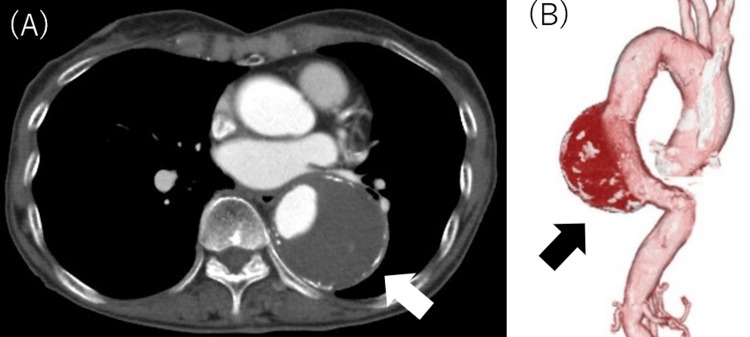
Preoperative contrast-enhanced CT. (A) Short-axis image revealing a 60 × 52 mm aneurysm with an abundant mural thrombus (white arrow). (B) A 3D reconstructed image revealing an aneurysm in the descending thoracic aorta in the region from Th8 to Th11 (black arrow). The image is visualized from a dorsal perspective.
